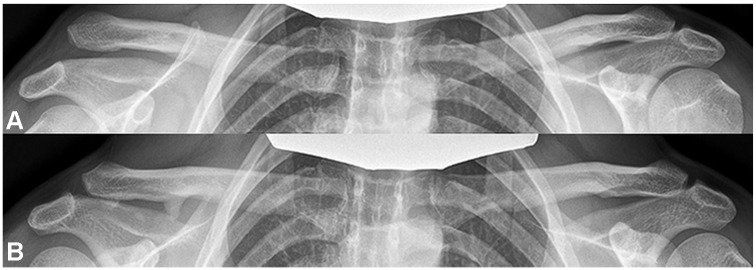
(A) Panoramic radiograph with 10-kg load at the time of injury showing a Rockwood type 5 dislocation on the right acromioclavicular joint. (B) Panoramic radiograph with 10-kg load at final follow-up showing a spontaneous reduction of the injured acromioclavicular joint after nonoperative treatment.
